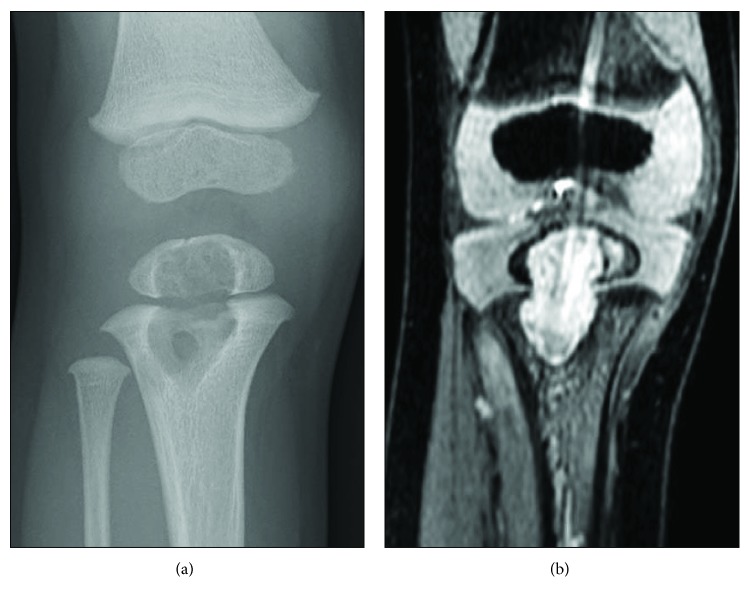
After treatment with intravenous antibiotic chemotherapy. (a) Plain radiograph showing the epiphyseal radiolucent lesion extending to the metaphysis through the physis at the right proximal tibia. (b) MRI demonstrating an abnormality at the same site, extending across the high-signal intensity area of the physis in the three-dimensional fast field echo sequence.
